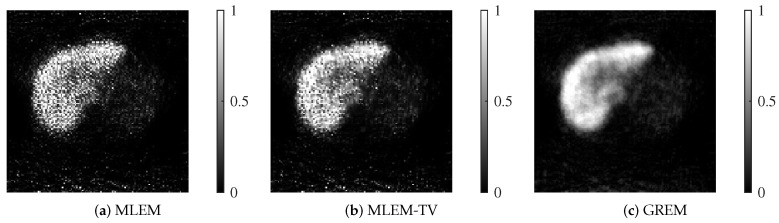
Reconstructed images of MLEM, MLEM-TV, and GREM with ^99m^Tc-GSA liver SPECT data: (a) MLEM, (b) MLEM-TV, (c) GREM.
